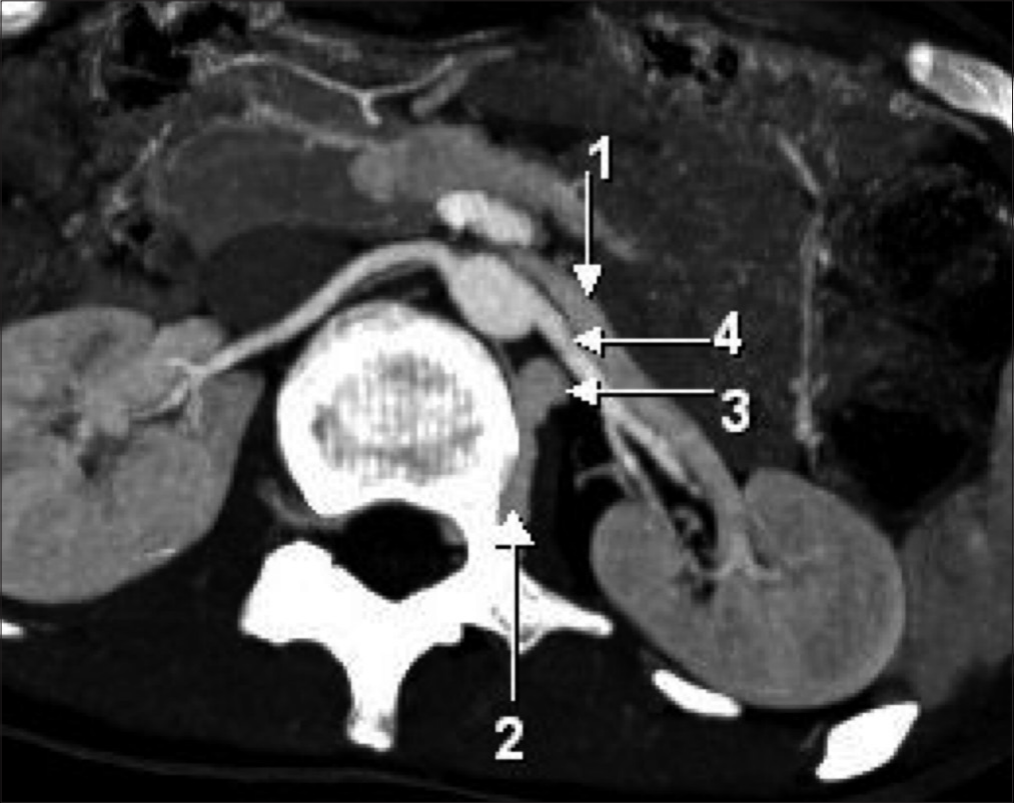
Axial maximum intensity projection CT scan shows the left renal vein, the left renal artery, and the communicating vein as distinct structures. (The arrows point to the same structures as in Figure 3)
